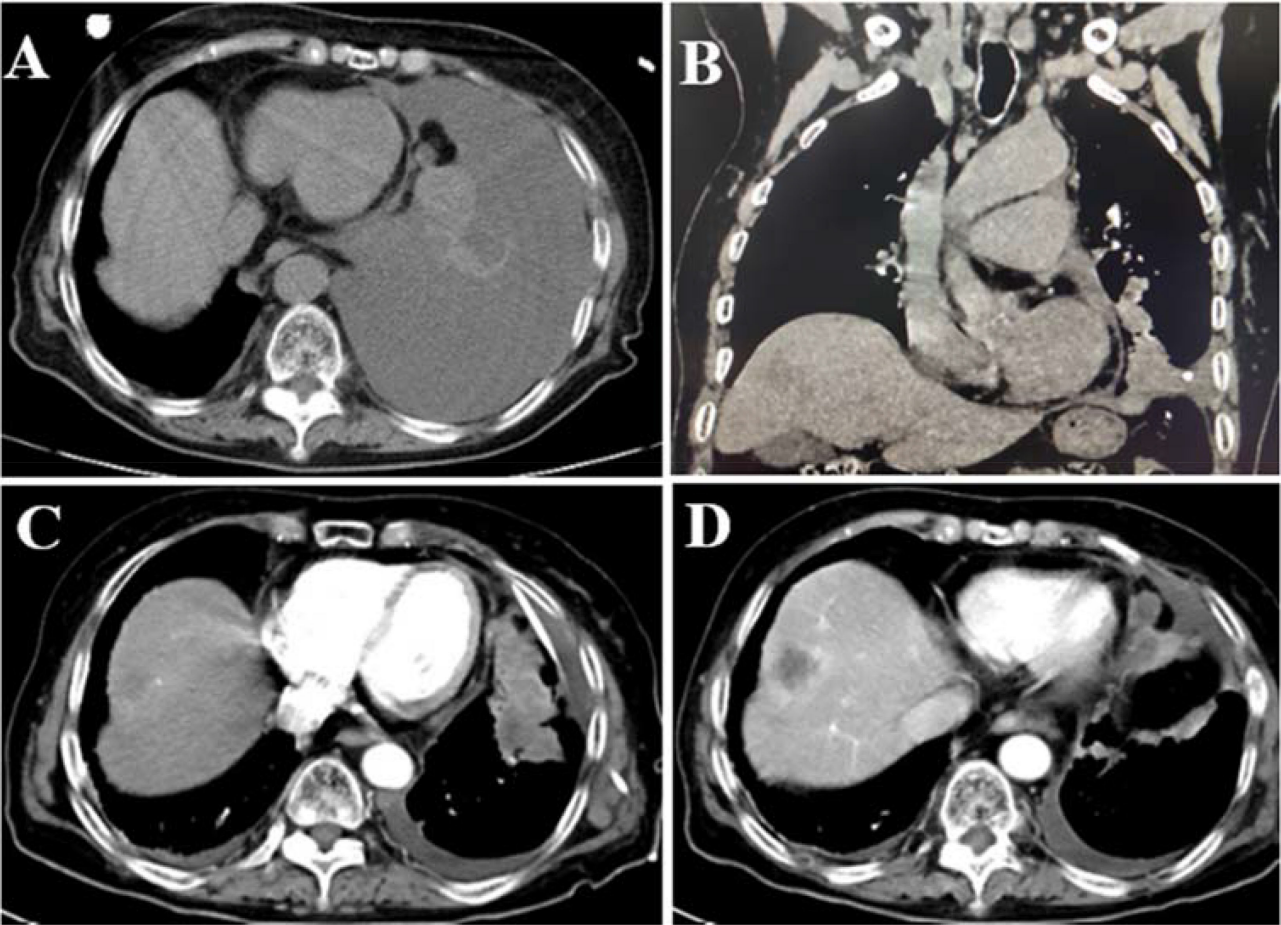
CT images of the reported case. (A) Massive left pleural effusion was observed during the initial visit. (B–D) Enhanced CT scans of the chest after pleural effusion drainage clearly demonstrated both the lung tumor, pleural metastasis and "bull's-eye" hepatic metastases.
